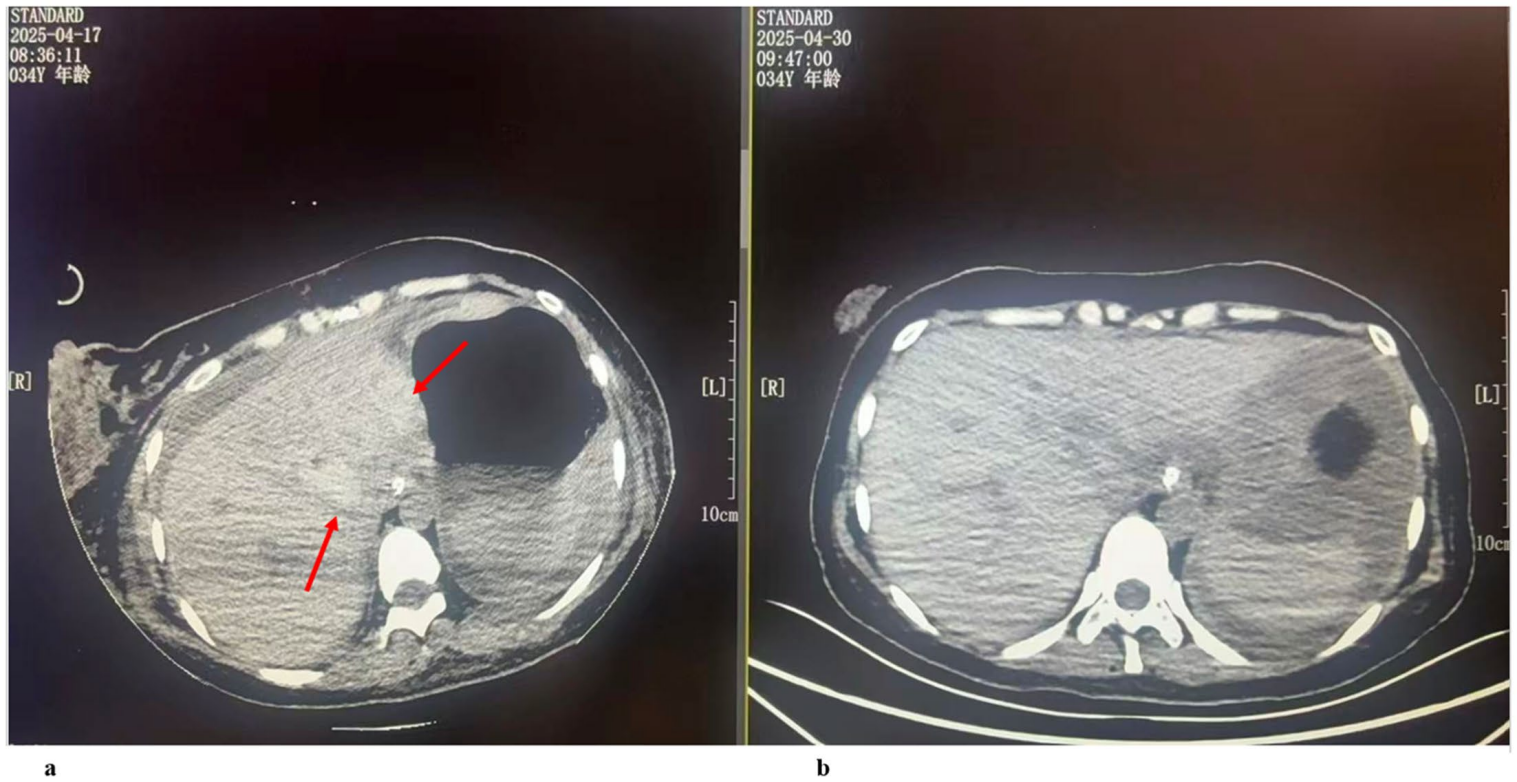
(a) On postoperative day 5, abdominal CT of the patient revealed hepatic hemorrhage (indicated by the arrow). (b) On postoperative day 18, the patient’s abdominal CT showed that the hemorrhage had been resorbed compared with previous images.
